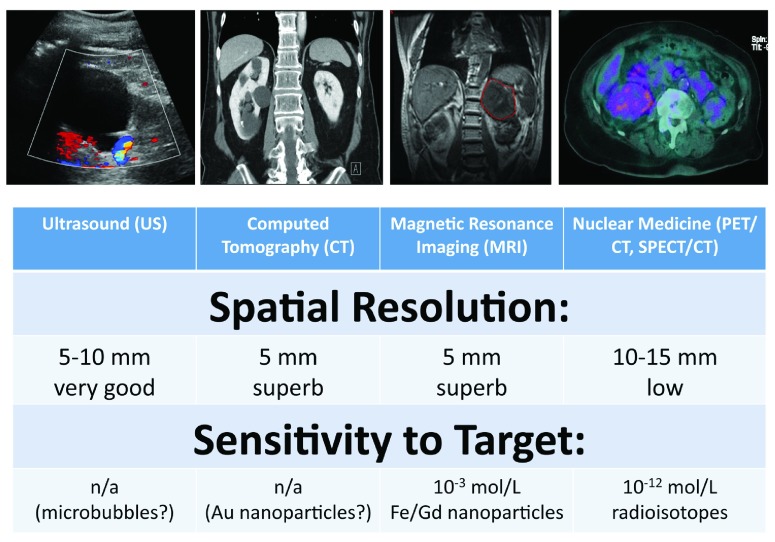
Comparison of different molecular imaging modalities. The anatomic spatial resolution and sensitivity for different molecular imaging methods are shown. CT and MRI based methods have excellent anatomic resolution, whereas PET/CT and SPECT/CT have very high sensitivity for detecting molecular targets.
